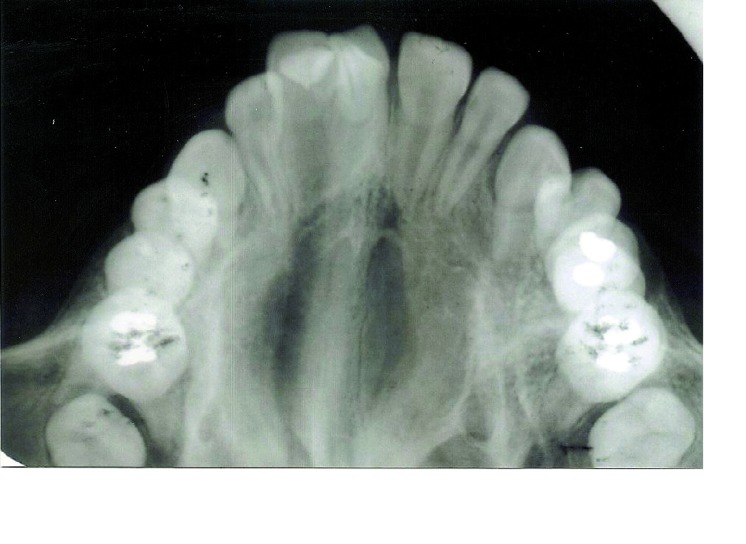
Occlusal radiograph depict central incisor fused along the entire length to the supernumerary tooth. Each tooth had a separate pulpal system and roots.
